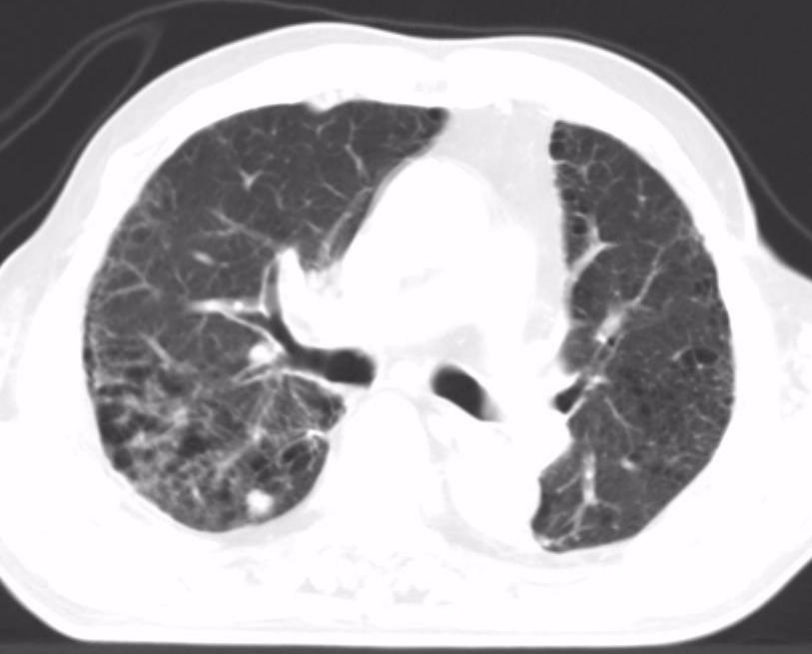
CT images before SBRT (Pt. No. 5).
